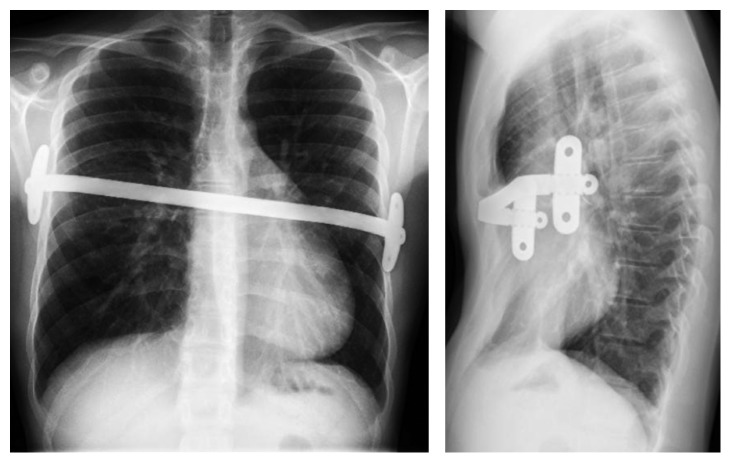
Anteroposterior view and lateral view of thoracic X-ray two years after the insertion of the Nuss bar.
